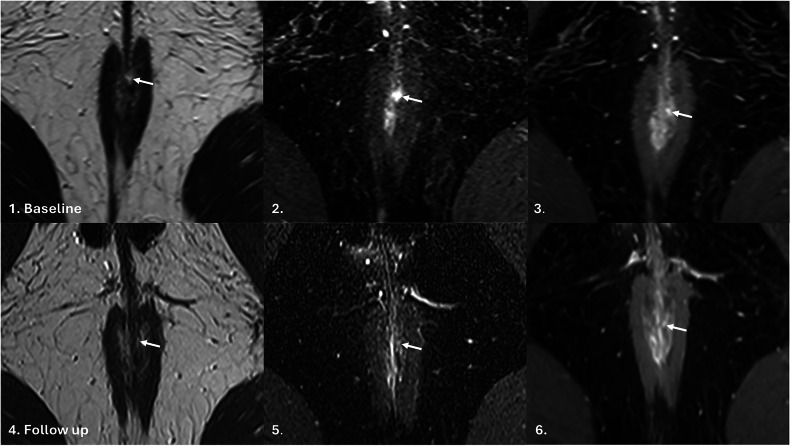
TOpClass-radiologically improved fistula with clinical recurrence. From top to bottom, left to right: Baseline MRI. 1 Axial oblique T2-weighted, (2) fat-saturated T2-weighted and (3) post-contrast fat-saturated T1-weighted images demonstrate a hyperintense tract (arrow), consistent with ongoing fistula activity. Follow-up MRI (4–6) (9 months later) demonstrates a focal area of residual hyperintensity (arrow), most conspicuous on the post-contrast fat-saturated T1-weighted image, consistent with radiological improvement but incomplete healing
